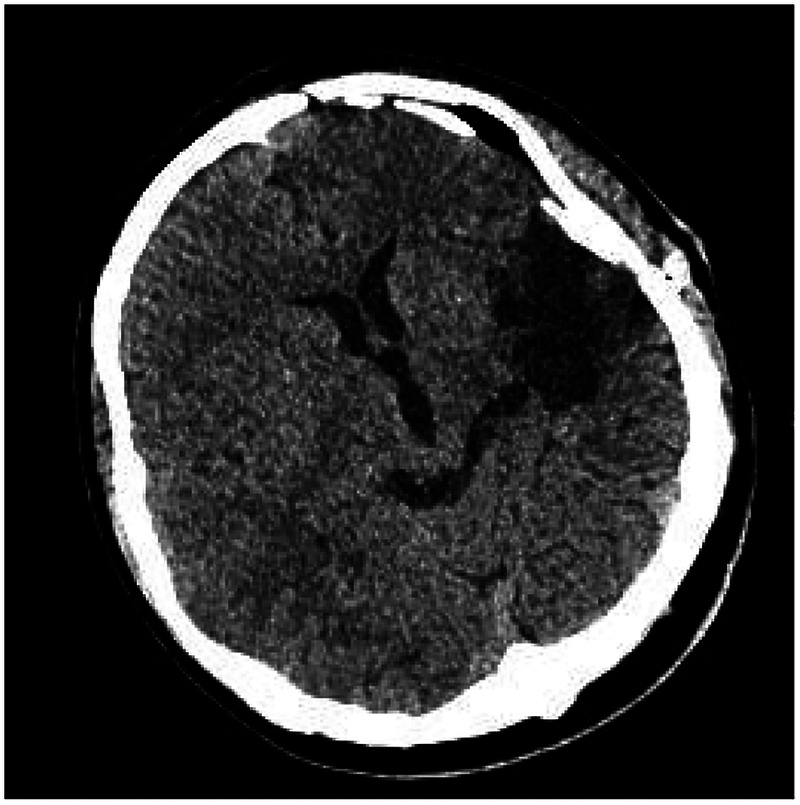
CT after cranioplasty demonstrates restoration of the cranial defect.
